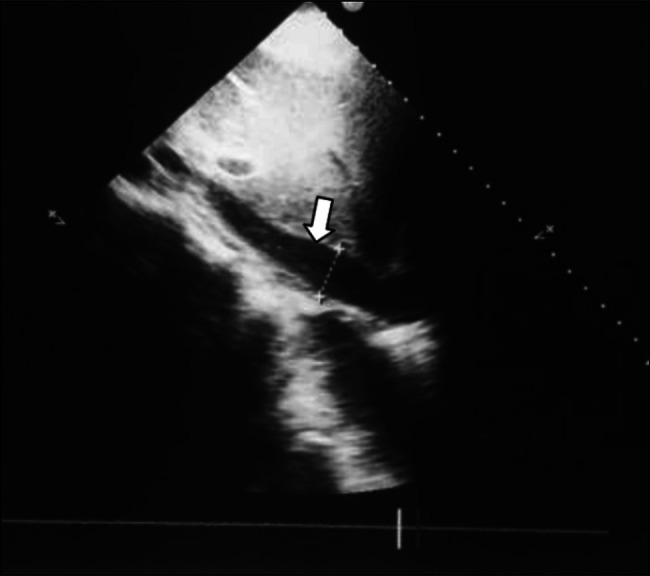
Echocardiogram showing thickening and pericardial effusion (white arrow).
